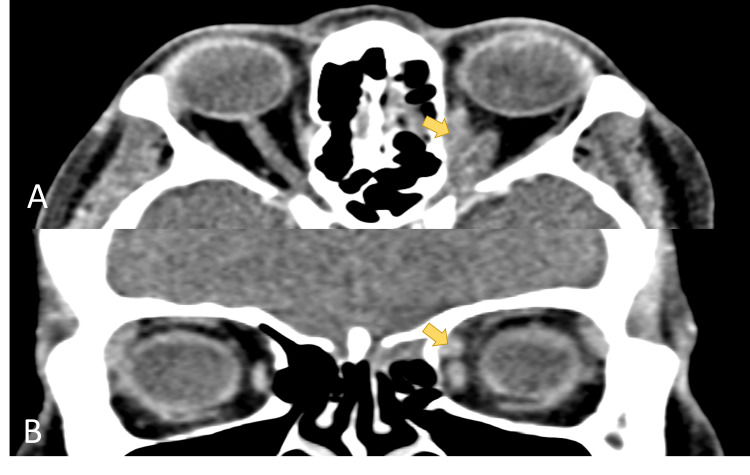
Computed tomography of the orbit during the first presentation. Axial view (A) and coronal view (B) of computed tomography of the orbit showed intraconal lesion (arrow) that extend superomedial and posteriorly involving the medial and superior rectus.
